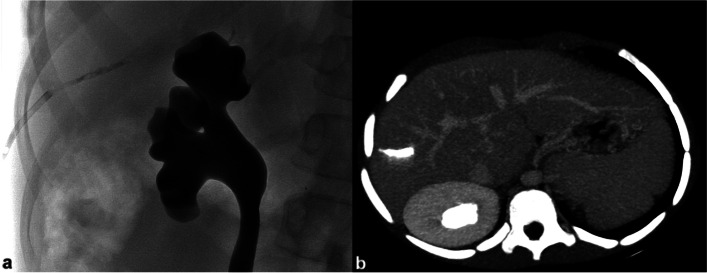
Embolisation of transhepatic tract with cyanoacrylic glue after PPVC. Fluoroscopy image shows the deployment of cyanoacrylic glue in the hepatic parenchyma tract, through a 4F introducer sheath during its retraction (a). MIP reconstruction of contrast-enhanced CT performed after the procedure displays the location of glue cast in the hepatic parenchyma and confirms the adjacent segmental portal branches patency (b)
